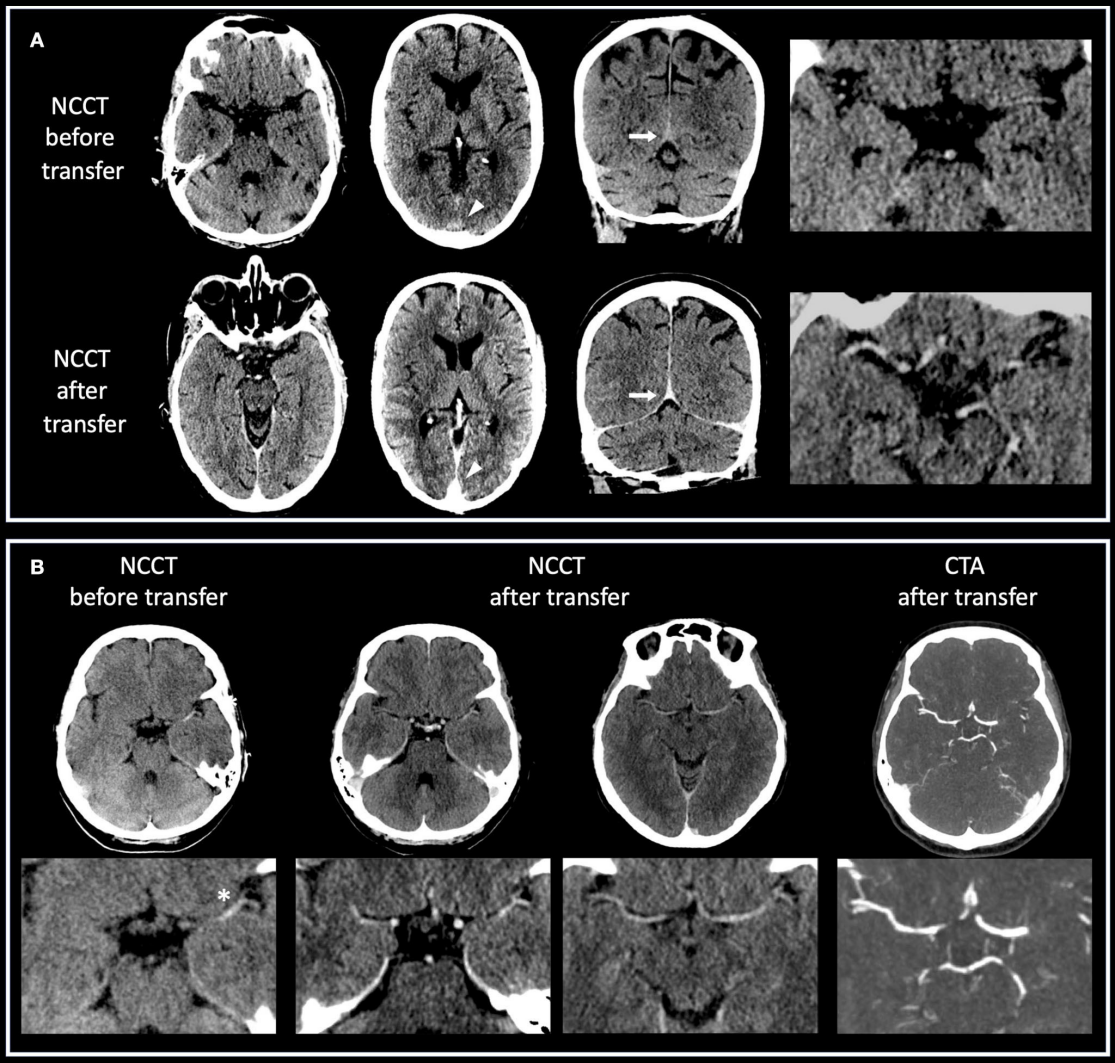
(A,B) Case examples of non-contrast CT imaging before and after interhospital transfer. (A) Patient with right-sided ICA occlusion who underwent CT imaging at PSC including CTA. Transfer time was 85 min, and kidney function was normal. Residual IV iodine contrast produces hyperdense intracranial vessels after the transfer, especially around the Circle of Willis (axial and enlarged views) and in sinuses on axial (arrowheads) and coronal (arrows) views. (B) Patient with left-sided M1 occlusion who underwent CT imaging at PSC including CTA. Transfer time was 90 min, and kidney function was normal. Presented are axial and enlarged views. Before transfer, a hyperdense vessel sign (asterisk) due to thrombotic occlusion is clearly visible on non-contrast CT. After transfer, intracranial vessels are hyperdense due to residual IV iodine contrast, which obscures intravascular thrombus. CTA after transfer confirms persistent vessel occlusion.
